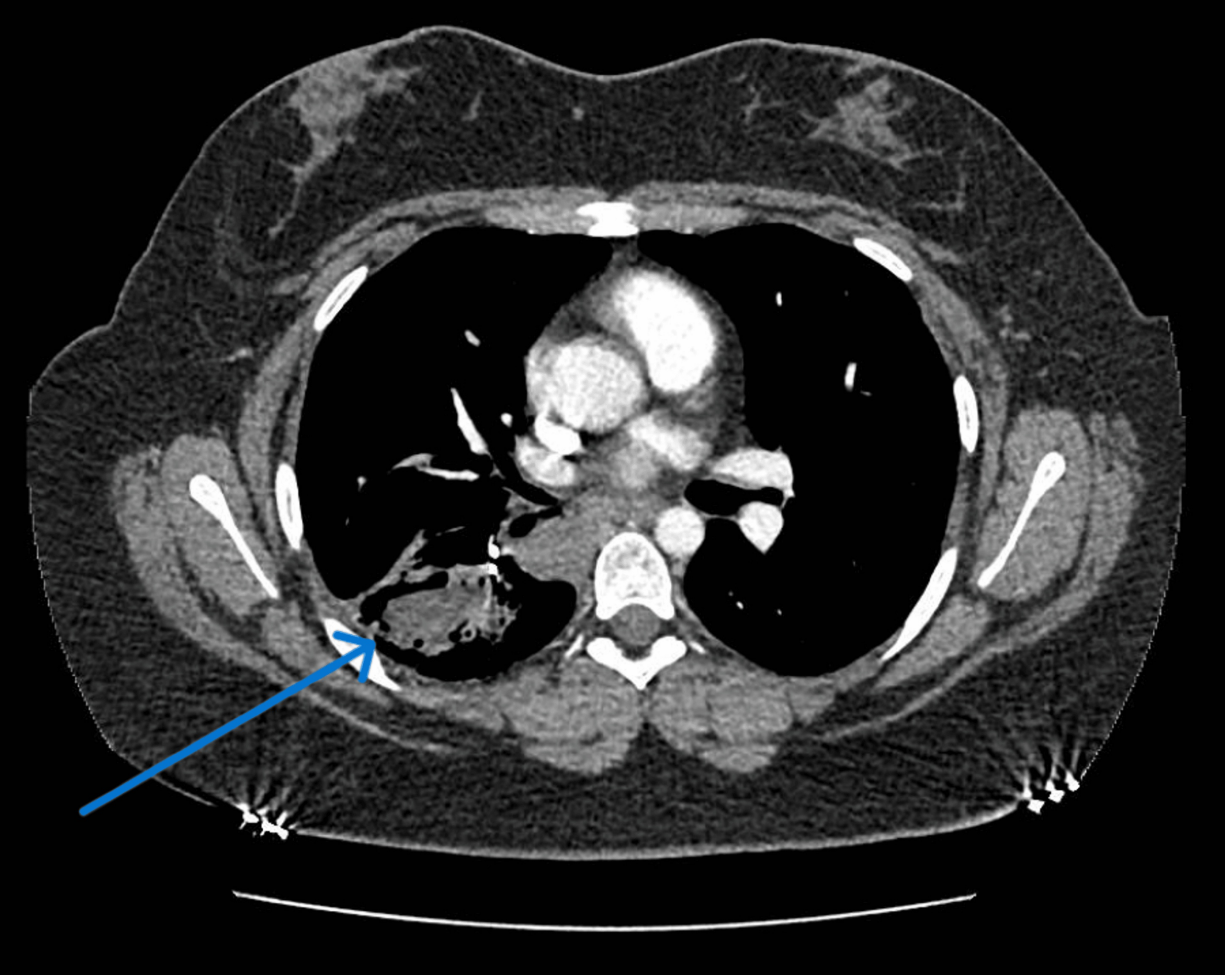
Computed tomography (CT) thorax showing a small fungus ball with an air crescent sign in the right lower lobe.
